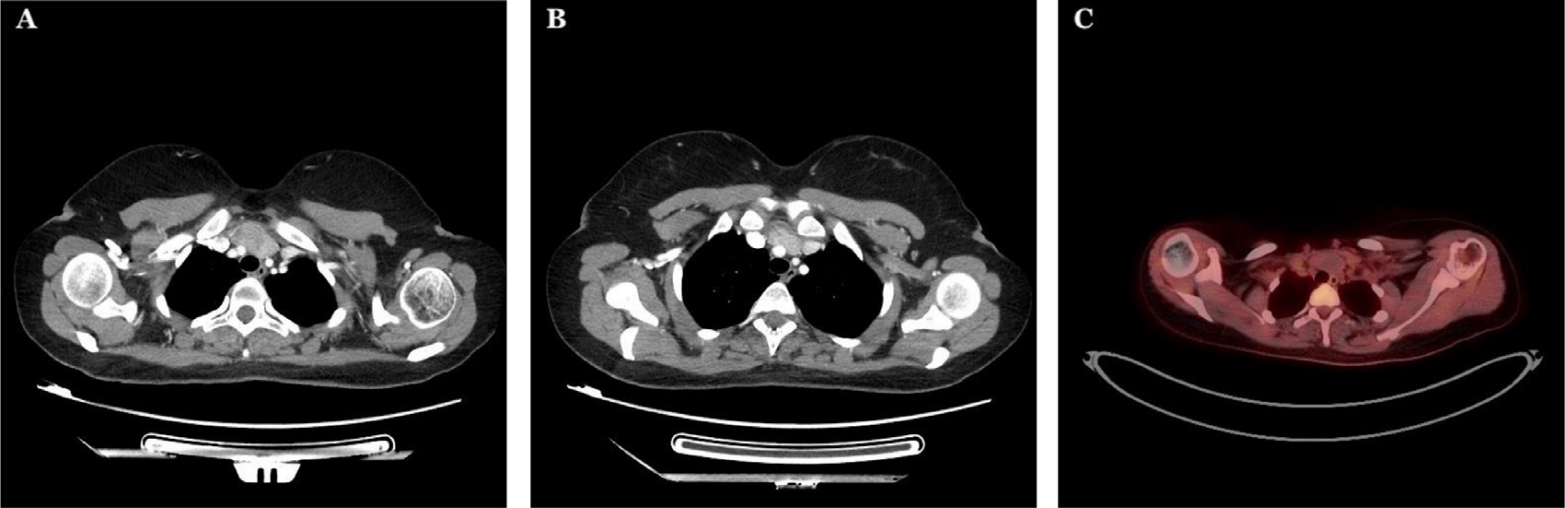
An axial CT showing part of the mass that is attached to the superior vena cava and innominate artery (A and B). An axial PET scan mass showing no observed uptake or metabolic activity (C).
